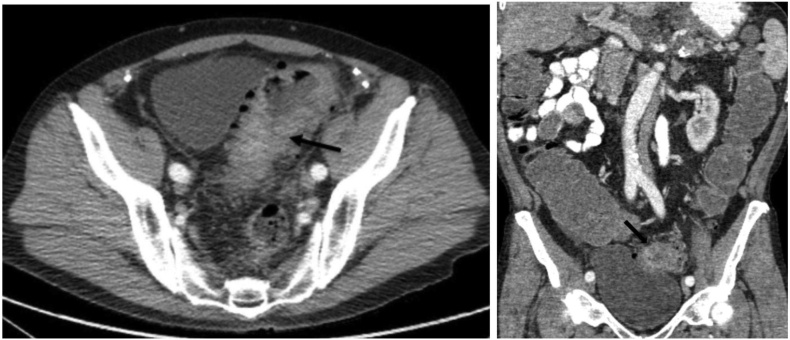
Axial (left) and coronal (right) CT scan - sigmoid mass (black arrow).
